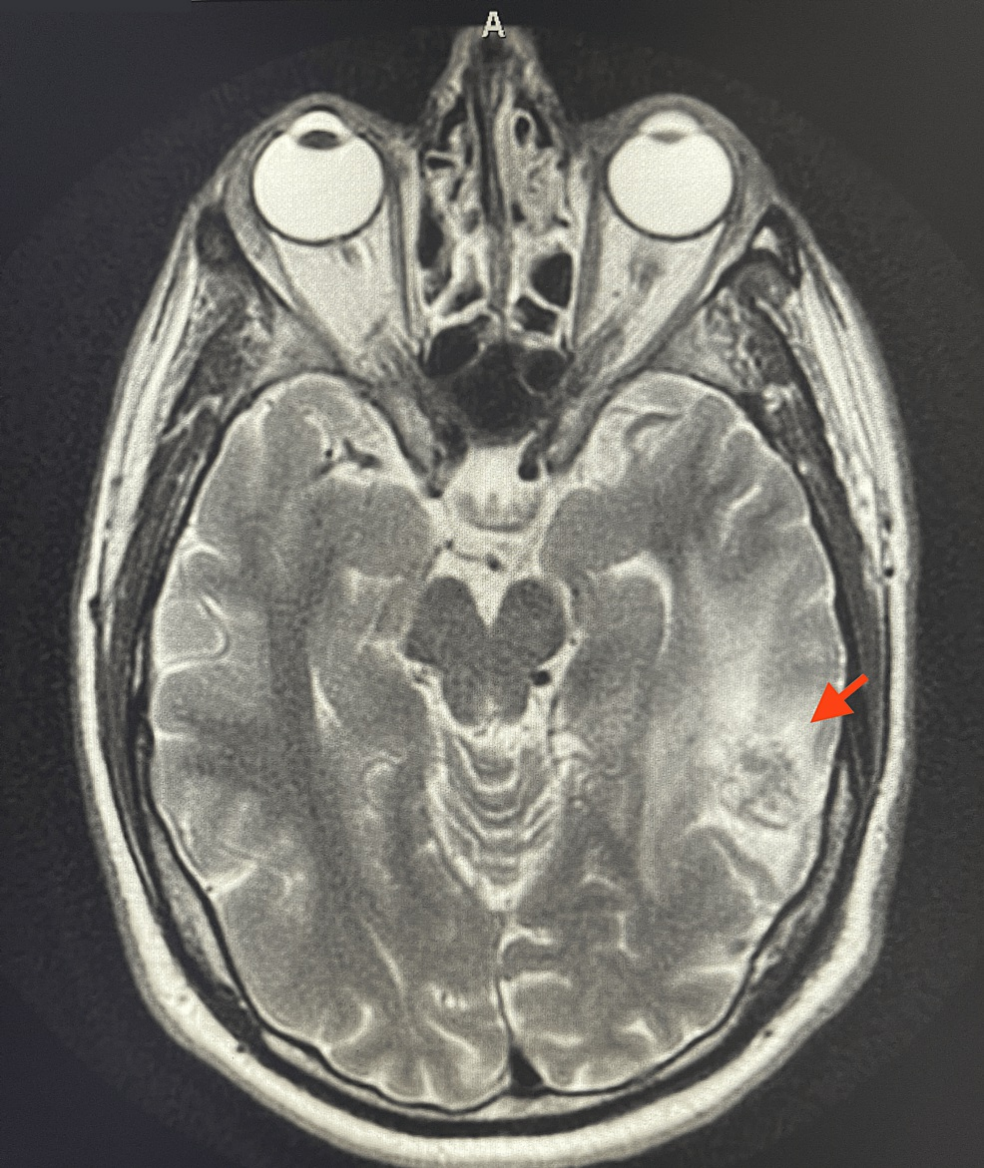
MRI with contrast performed during admission The MRI with contrast shows an AVM status-post radiation treatment in the left temporal lobe, as well as left temporal peri-lesional edema and mass effect of this area (red arrow).
